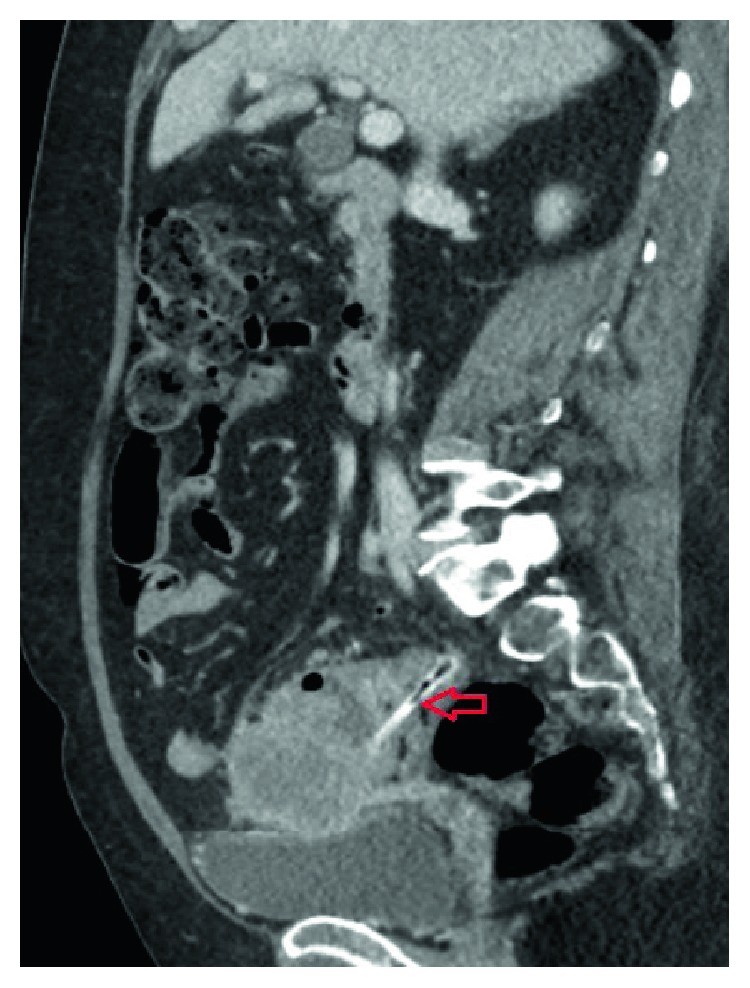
Sagittal CT highlighting a hyperdense tubular foreign object in the sigmoid colon (arrow), in addition to displaying a mass-like thickening of the sigmoid colon.
